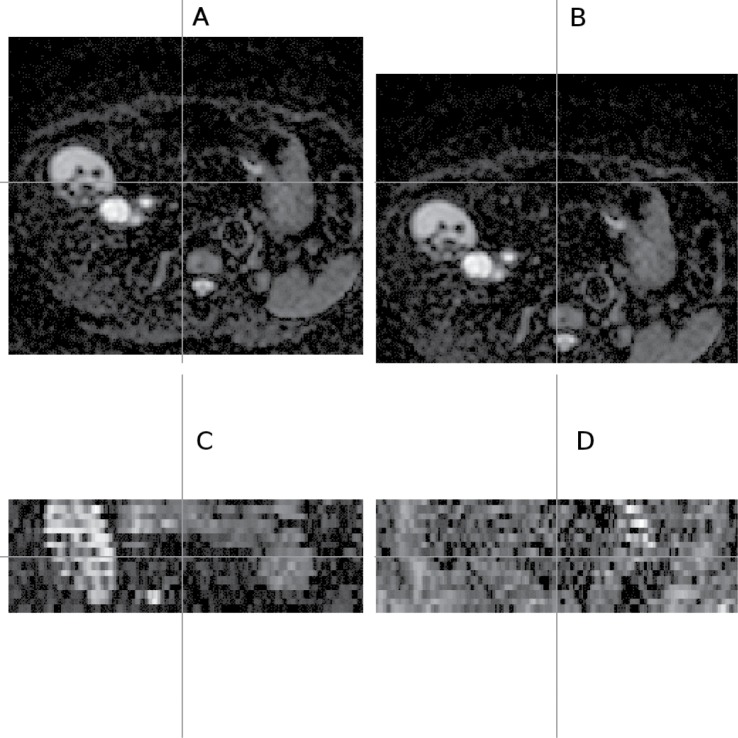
Odd-even effect observed in DW-MR data. Healthy liver DW-MR image (v3-0528) showing the odd-even effect (in the coronal view) as a result of an interleaving acquisition protocol; the coronal view images (C and D) show what is seen from the cross section of the horizontal line drawn on the transverse view slice (A and B); the two columns show different cross sections on the same volume; image volume selected is one of the repeat data-sets (out of 4) with diffusion gradient in −*x* direction; b-value = 100*s*/*mm*
